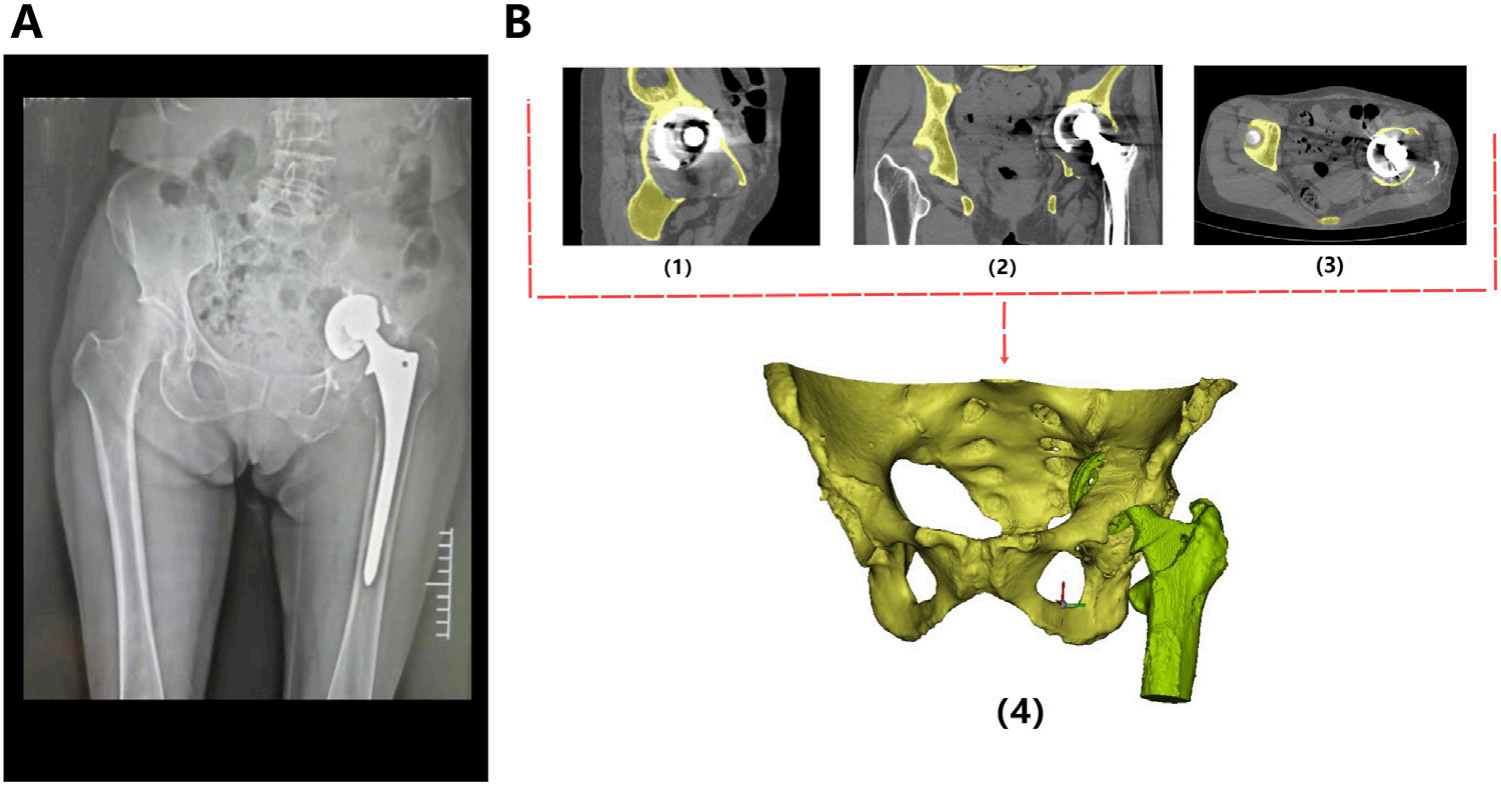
Preoperative radiological image of the patient. (A) The X-ray revealed loosening and dislocation of the acetabular cup. (B) CT scan images of the bilateral hip joints in sagittal (1), coronal (2), and axial (3) planes. Based on the CT data, a three-dimensional reconstruction was performed using MIMICS software (4), which stereoscopically demonstrates the morphology of the pelvis and proximal femur, as well as the location of the implants.
